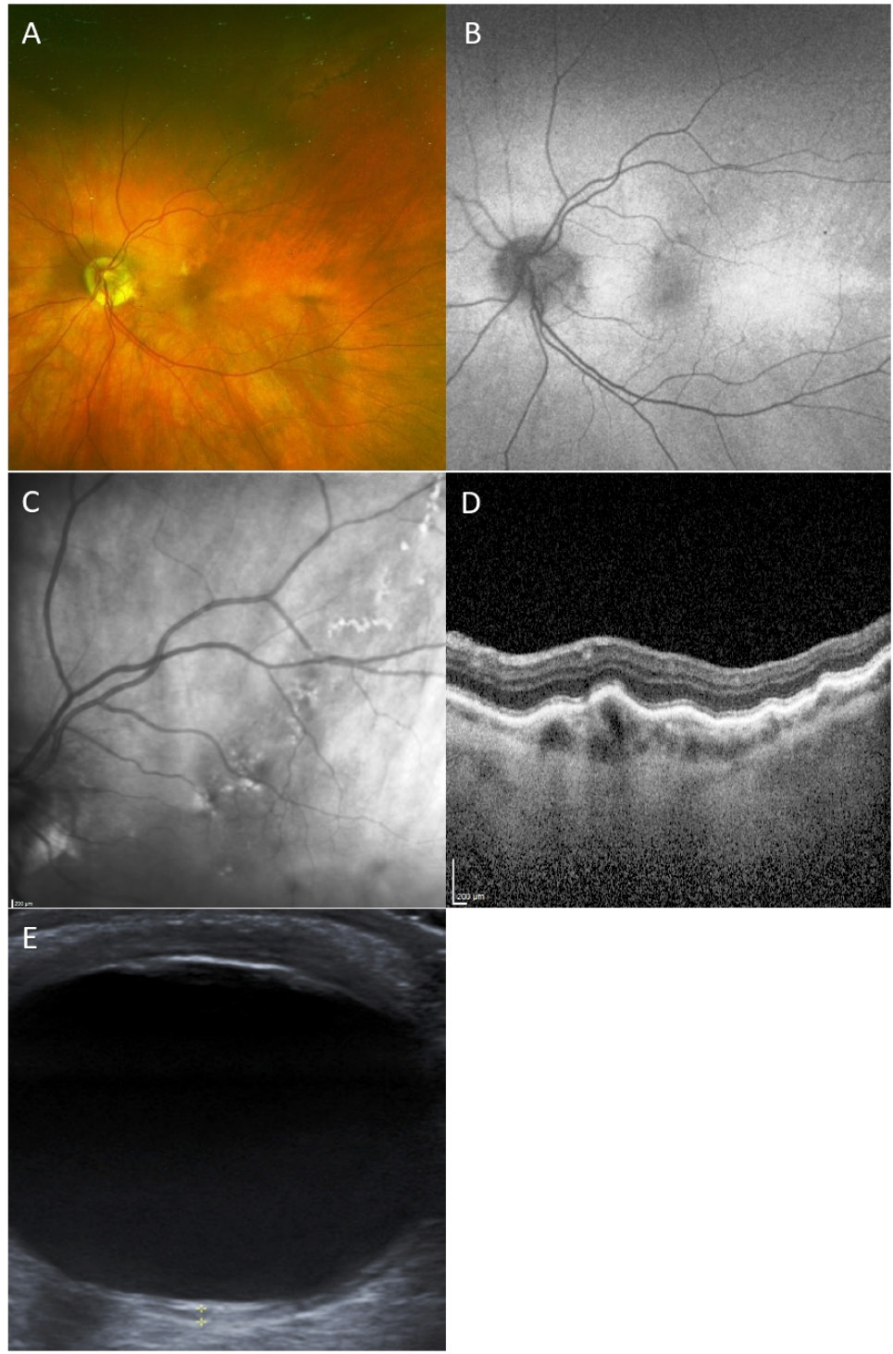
Pseudocolour fundus image, FAF, near-infrared image, EDI-OCT and B-scan us of a patient with type II CM. (A) Pseudocolour image shows RPE mottling in a track-like fashion. (B) FAF shows a hyperfluorescent and hypoautofluorescent track. (C) Near-infrared image shows hyper-reflectivity and hyporeflectivity. (D) EDI-OCT shows a hollow elevated choroidal area, occupying the entire choroid that is focally thickened. (E) On B-scan US, the CM presents as a nodular lesion with low internal echogenicity. CM, choroidal macrovessel; EDI, enhanced depth imaging; FAF, fundus autofluorescence; OCT, optical coherence tomography; RPE, retinal pigment epithelium; US, ultrasonography.
